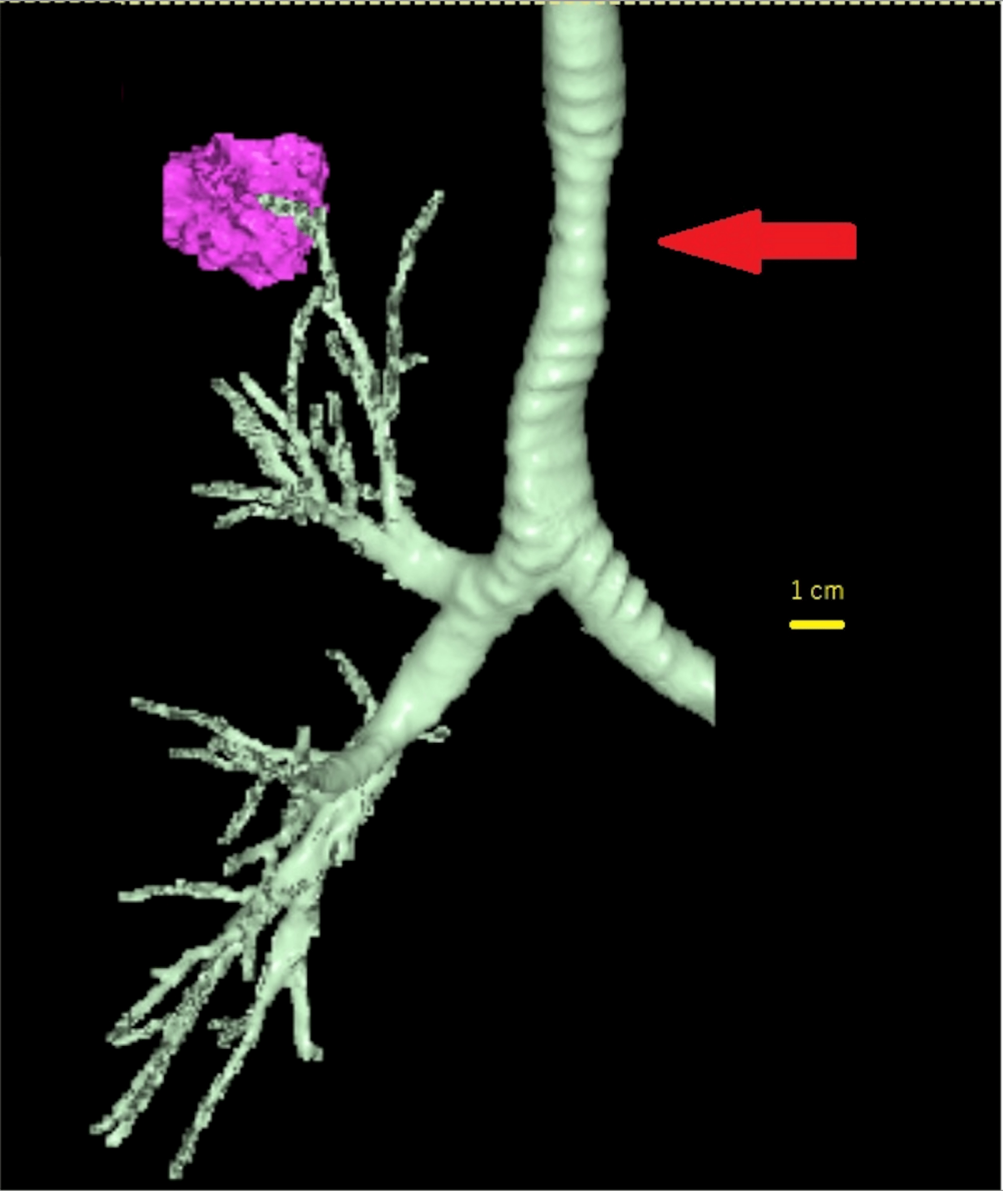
3D image constructed from a chest CT scan. The red arrow indicates the tracheal stenosis site, which spans approximately 2 cm in the cephalocaudal direction.
